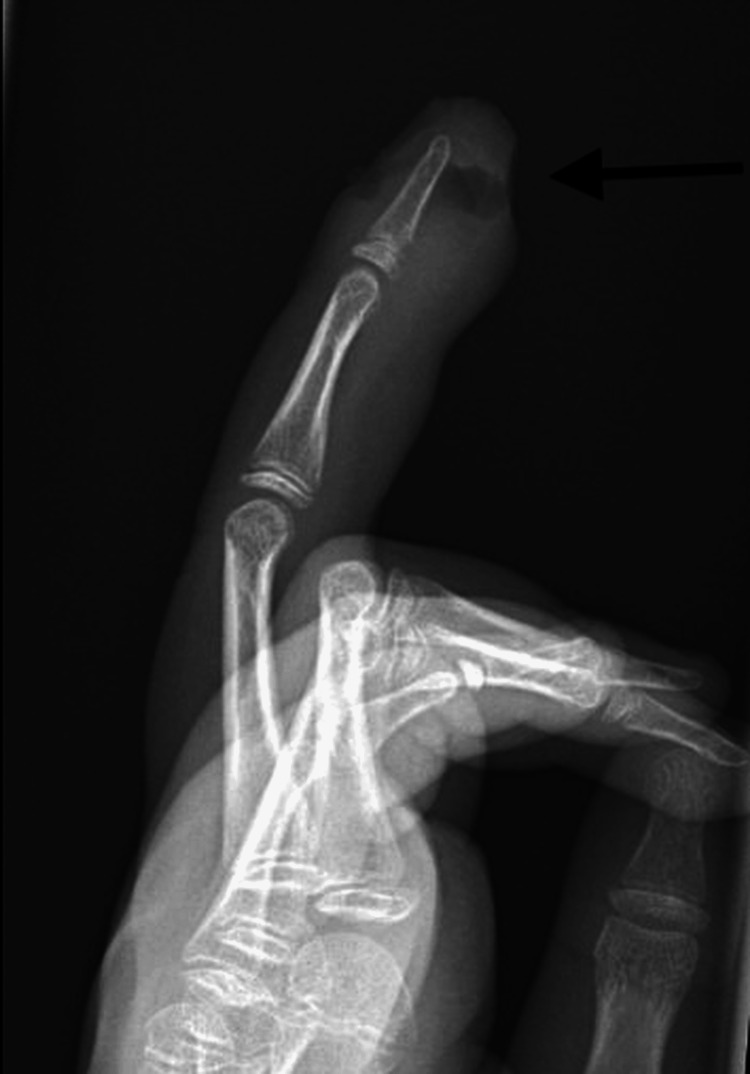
Lateral radiograph of the left third digit demonstrating distal soft tissue defect Lateral plain radiograph of the left hand demonstrating a soft tissue defect involving the distal aspect of the left third digit (arrow). No acute fracture or dislocation is identified on this view.
